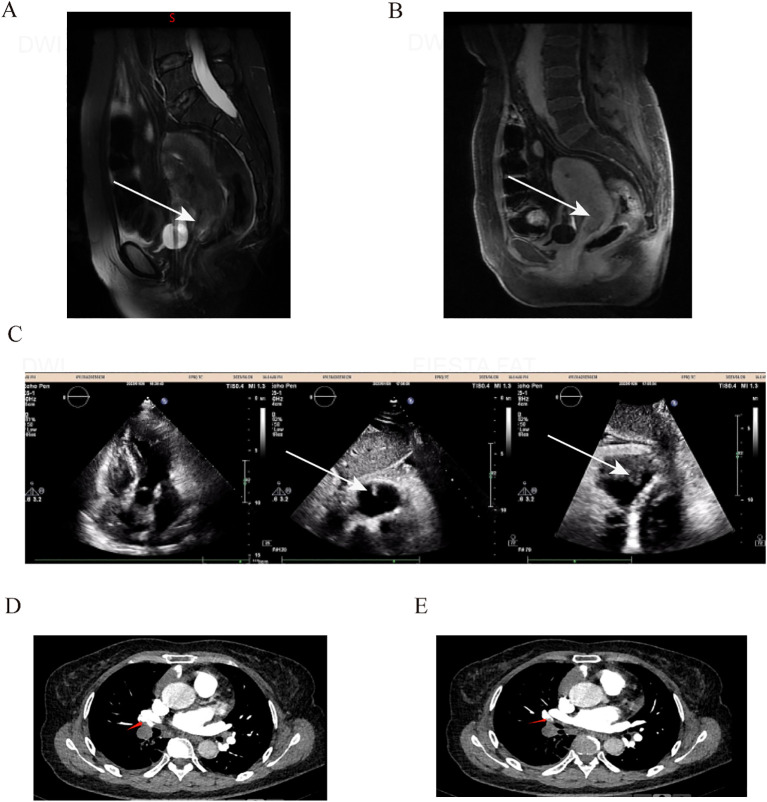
Magnetic resonance, echocardiography images and computed tomography pulmonary angiography. Figure 1. Magnetic Resonance and Echocardiography images. (A, B) T2 and Cor LAVA sequences of contrast-enhanced pelvic MRI showing the cervical lesion. (C) Transthoracic echocardiogram image. The white arrow indicated the lesions. (D, E) Computed tomography pulmonary angiography. The red arrow indicated a low-density filling defect in the pulmonary artery of the right lower lobe and its distal branches.
